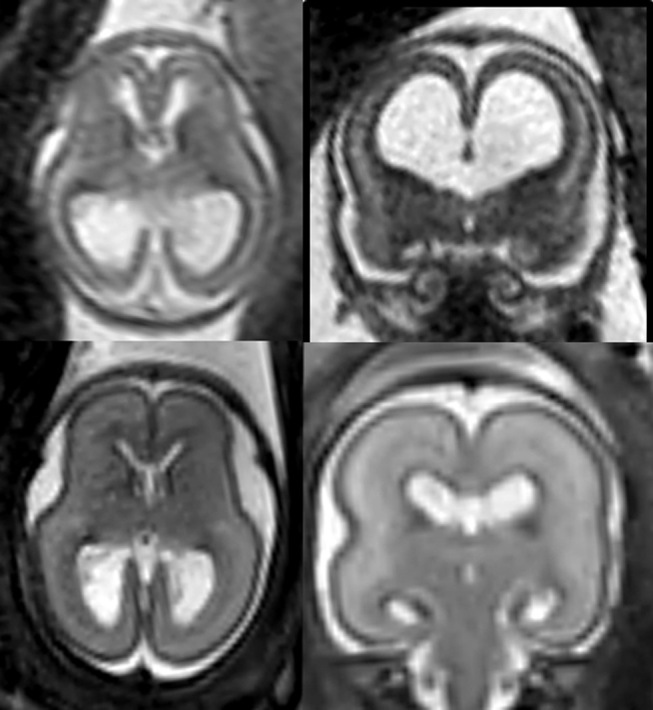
Fetal MRI in a case of Witteveen-Kolk Syndrome (OMIM: 613406) at 18+2 (upper row) and 23+4GW (lower row). Initially severe ventriculomegaly was found at sonography and confirmed by MRI at 18+2GW. While ventricular width was normalizing at 23+4GW, there were several MR softmarkers indicating the presence of a more complex phenotype: Delayed opercularization and verticalization of hippocampi, thalamic fusion and fusion of the fornices.
